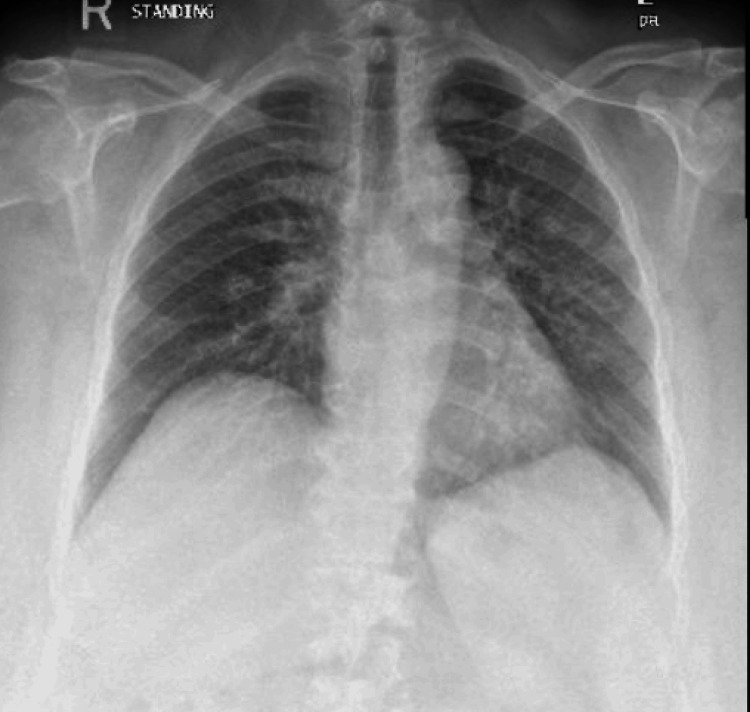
Chest X-ray showing grossly normal lung parenchyma with no obvious pulmonary pathology
